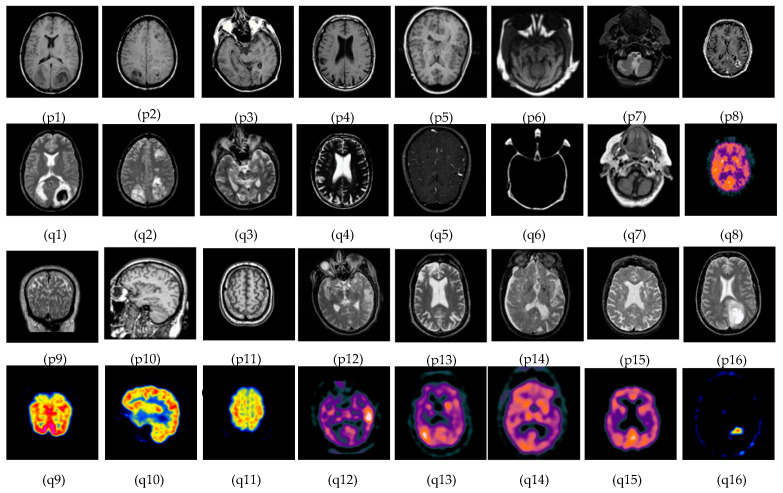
Medical image datasets: (p1–p4) and (q1–q4) are MR T1–MR T2 input images: (p5) and (q5) are T1 weighted MR–MRA input images; (p6,p7) and (q6,q7) are MRI–CT input images; (p8–p11) and (q8–q11) are MRI–PET input images; and (p12–p16) and (q12–q16) are the MR-T2–SPECT input images.
